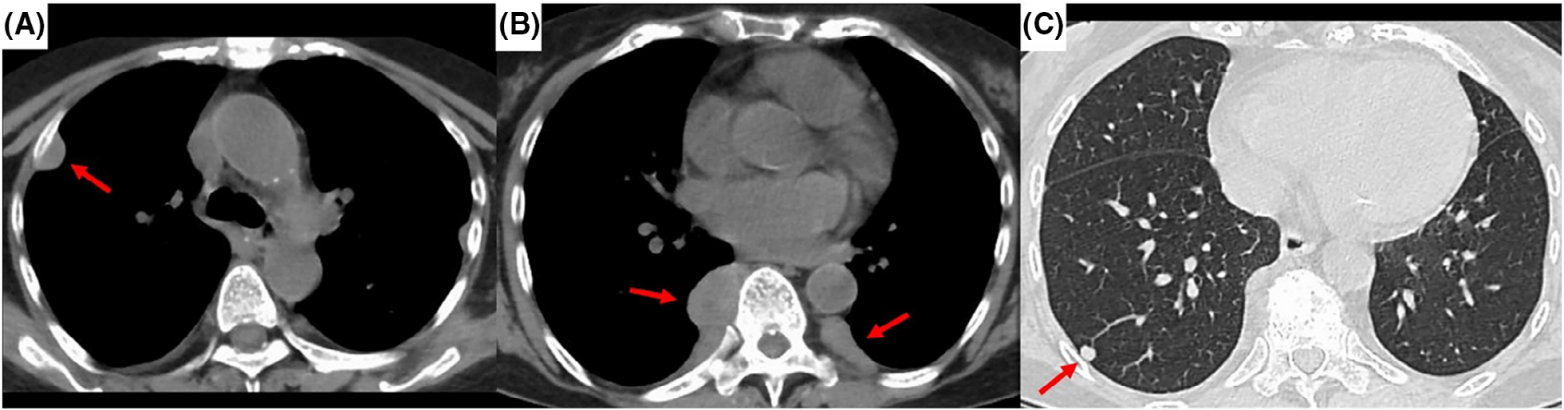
Images of chest computed tomography are shown. In the mediastinal window (A, B), bilateral pleural nodules are revealed. In the pulmonary window (C), a small solid lung nodule is revealed in right lower lobe.
